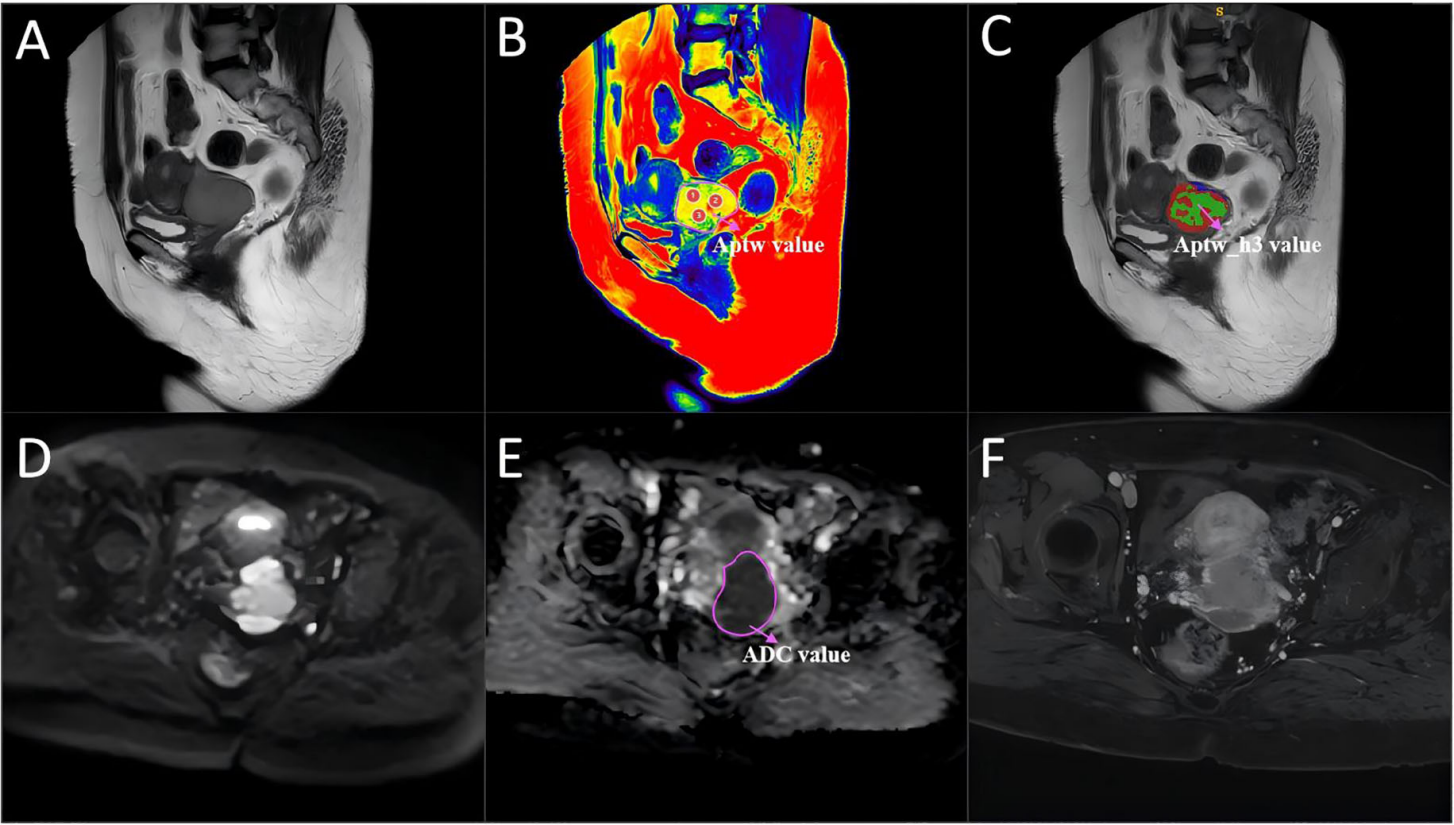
A 54-year-old woman with cervical cancer and LVSI, a serum CA125 level of 44.5 U/mL and a largest tumor diameter of 46 mm. (A) Sagittal T2WI. (B) APTw and T2W image fusion. (C) tumor region divided into three subregions with unsupervised clustering; red: subregion 1; blue: subregion 2; and green: subregion 3. (D) Diffusion-weighted image (b = 800 s/mm^2^). (E) ADC image. (F) DCE-MR image. The mean APTw and ADC values measured by the two radiologists were 4.36% and 0.83×10^−3^ mm^2^/sec, respectively. APTw, amide proton transfer-weighted; LVSI, lymphovascular space invasion.
